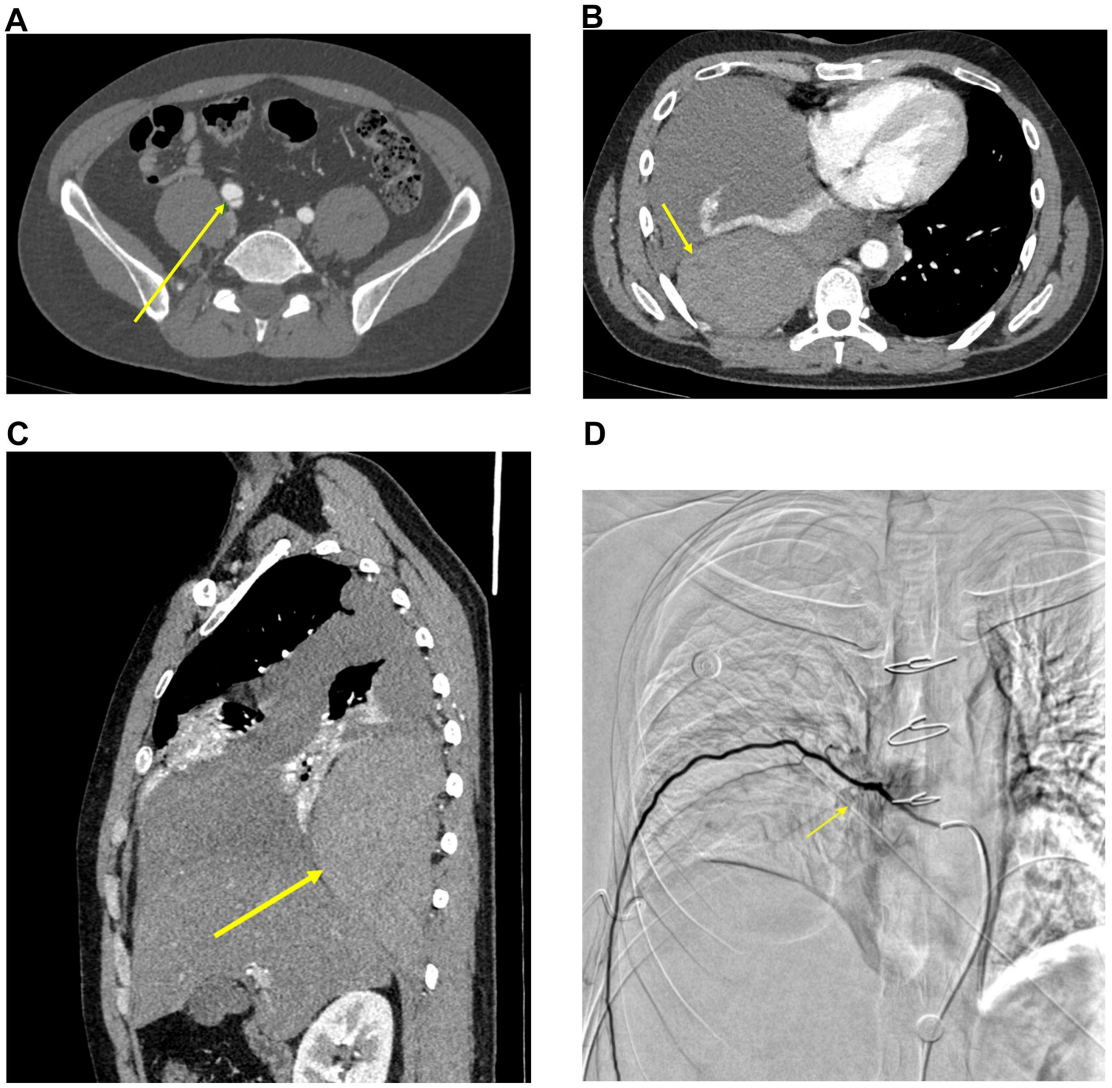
Serial imaging findings of a patient with vascular Ehlers-Danlos syndrome. (A,B) Axial contrast-enhanced CTA (November 5, 2024). (A) Right common iliac artery dissection (arrow). (B) Right extrapleural hematoma (arrow). (C) Sagittal contrast-enhanced CT (November 5, 2024), depicting the same right extrapleural hematoma (arrow). (D) DSA (November 8, 2024), showing percutaneous aortic and intercostal artery angiography. A dilated intercostal artery is visualized (arrow).
